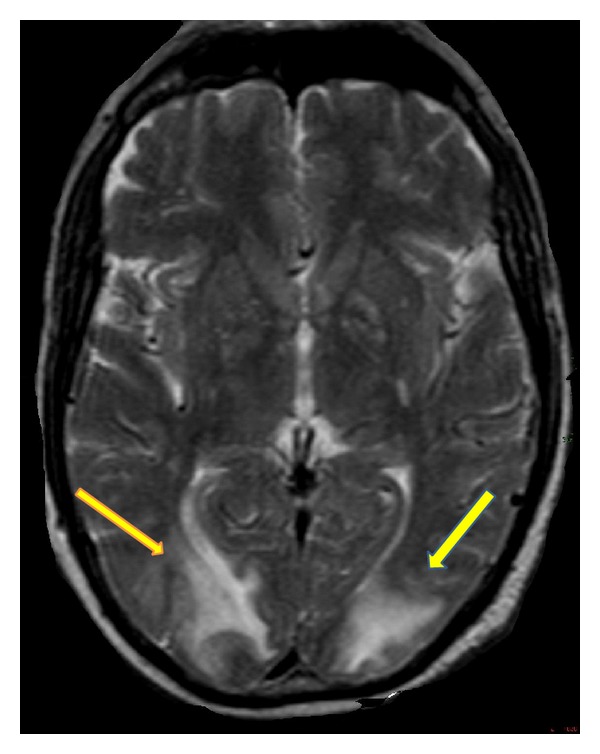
Increased fluid-attenuated inversion recovery (FLAIR) uptake sequence on cerebral occipital parietal regions.
